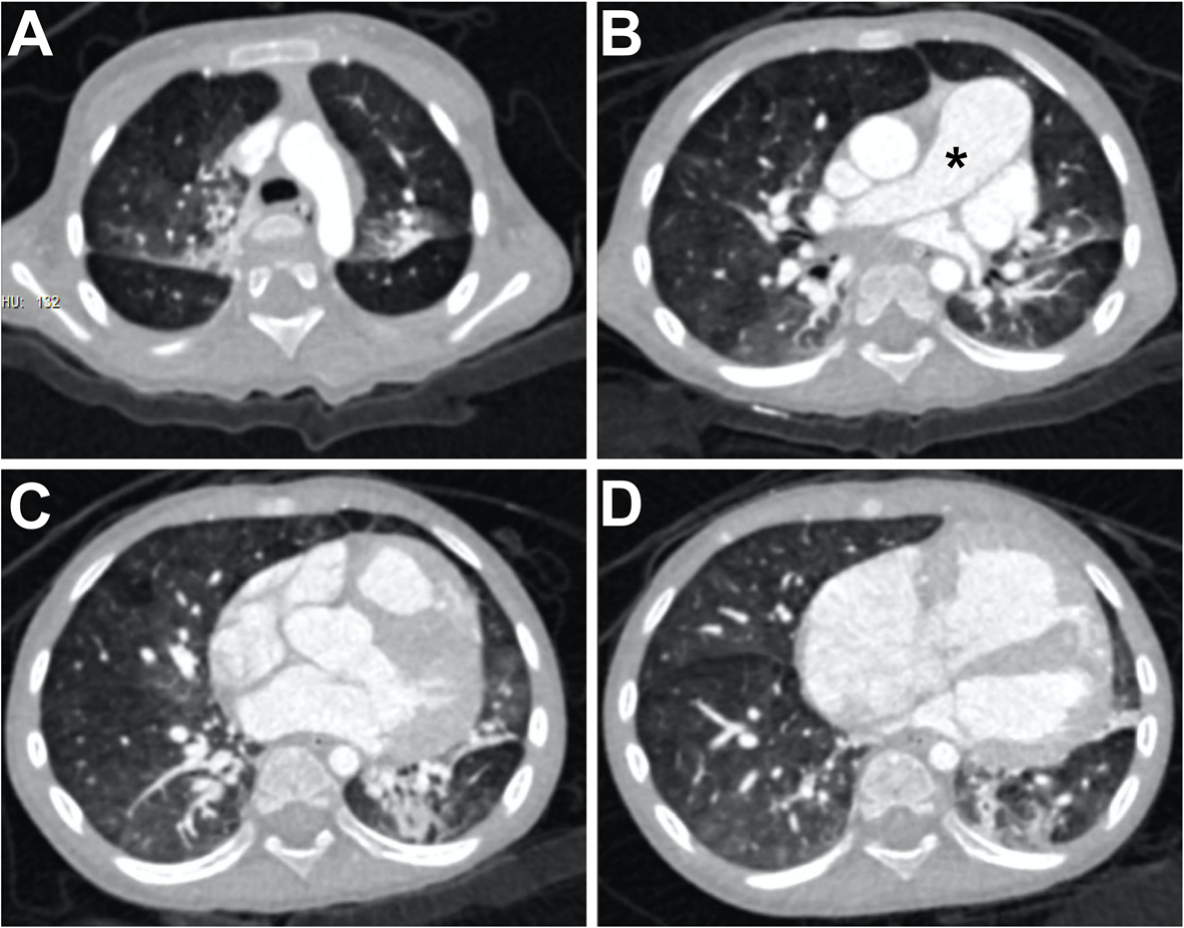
Chest CT. a Infiltration in both upper lobes of lung; b Main pulmonary artery was dilated (*). There was atelectasis in left upper lobe of lung; c Slightly infiltration in lower lobes; d Dilated right atrium.
